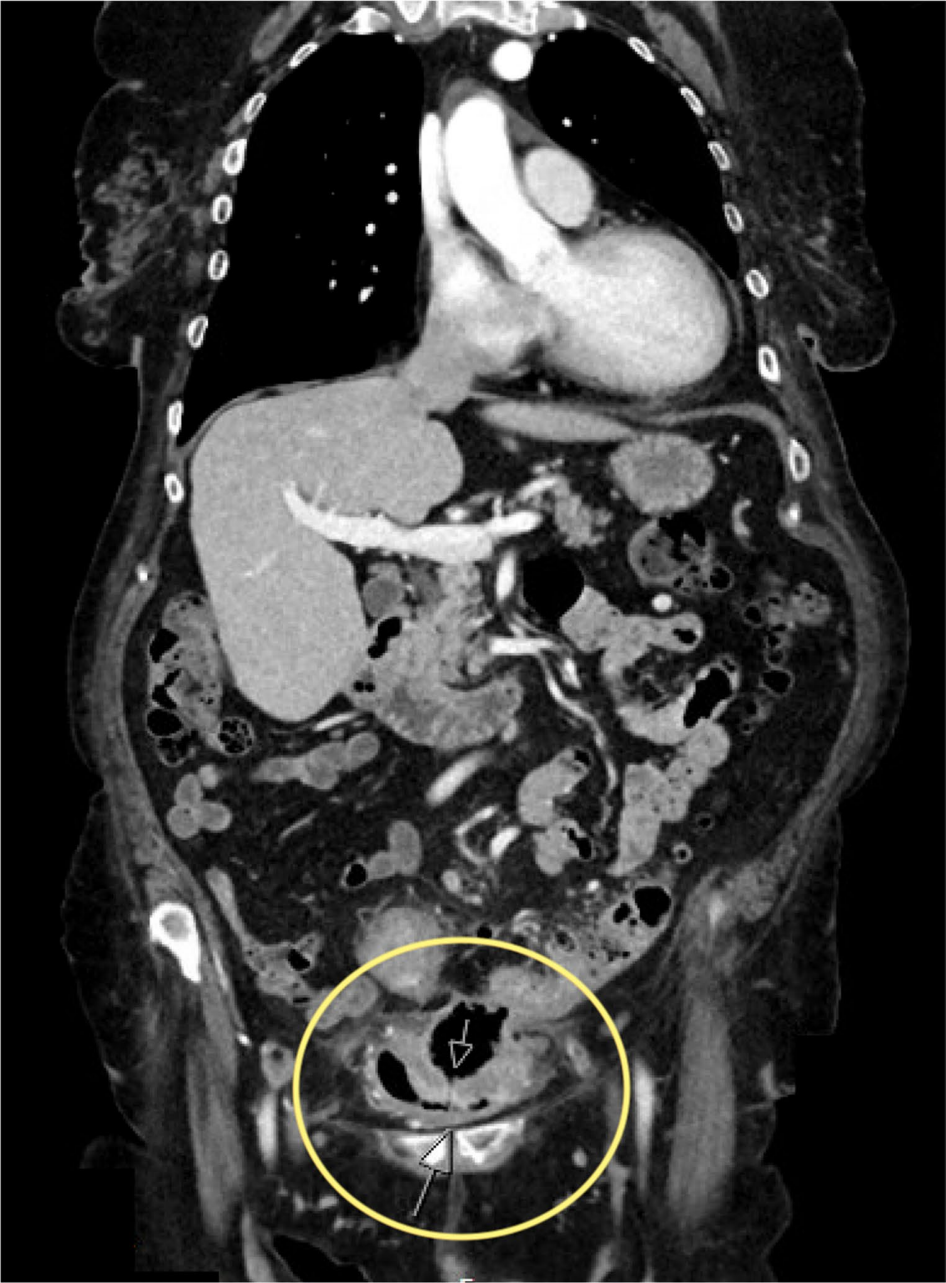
CT Imaging of a sigmoid bladder Fistula (SVF) highlighted by arrow
